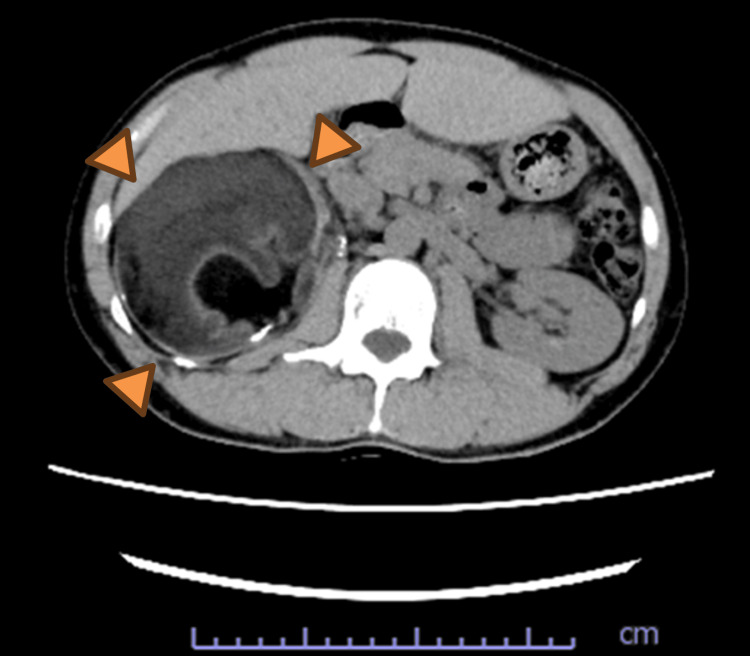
Abdominal CT findings CT shows a fat-containing mass measuring approximately 10 cm in diameter, located at the upper pole of the right kidney CT: computed tomography
